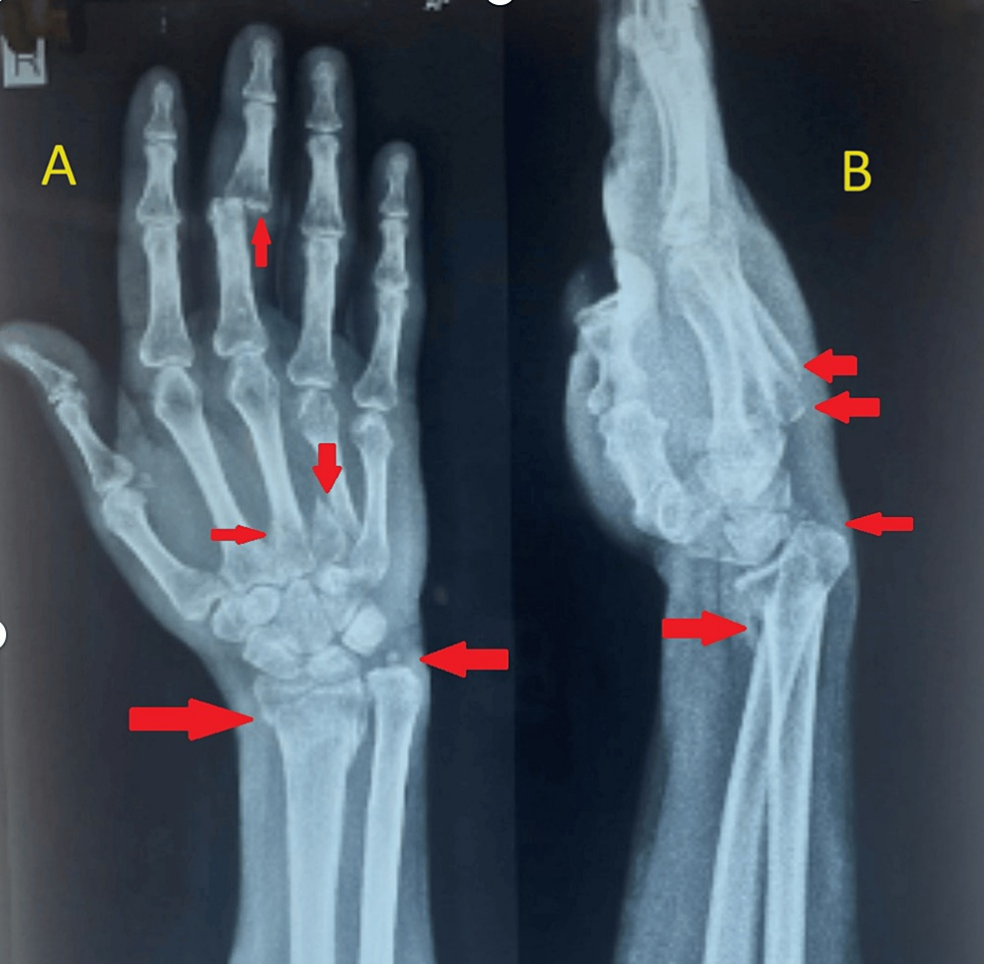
X-ray of the right wrist with hand anteroposterior (A) and lateral (B) views (A) shows the malunited distal end radius fracture with ulna styloid fracture and distal radioulnar joint disruption on the right side, the malunited base of the 4^th^ metacarpal fracture on the right side, the united 3^rd^ metacarpal fracture, and fracture dislocation of the proximal interphalangeal (PIP) joint of the 3^rd^ digit of the right hand. (B) shows the distal radioulnar joint disruption, positive ulnar variance, and malunited 3^rd^ and 4^th^ metacarpal fractures.
